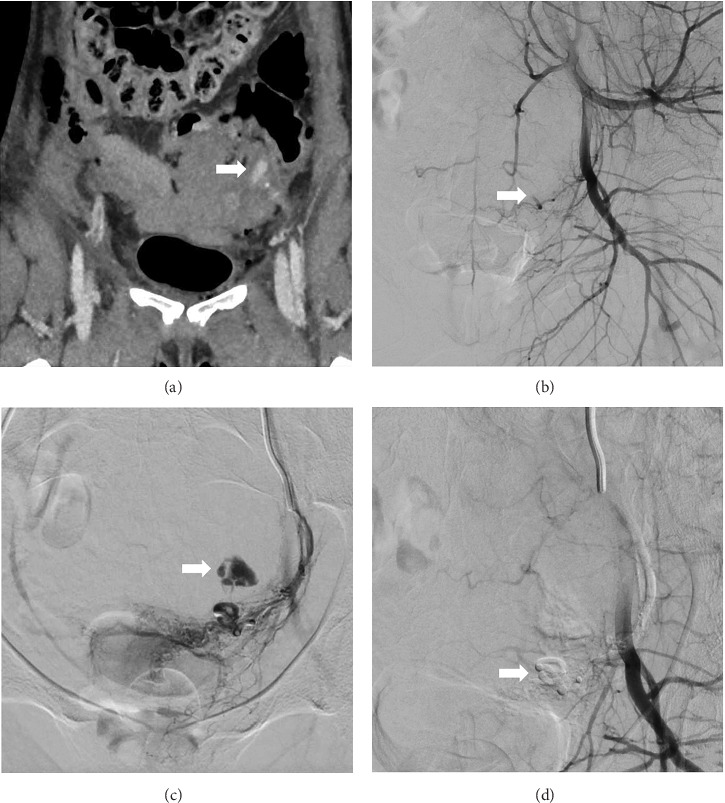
Case 8. (a) CT angiogram after bleeding from abdominal hysterectomy showing a left pelvic haematoma with active contrast extravasation, consistent with site of active bleeding (arrow). Angiograms show (b) selective catheterisation of the left internal iliac artery showing abrupt termination of the left uterine artery from vasospasm (arrow), (c) superselective catheterisation showing active bleeding (arrow) and (d) successful haemostasis following embolisation with Gelfoam (arrow).
